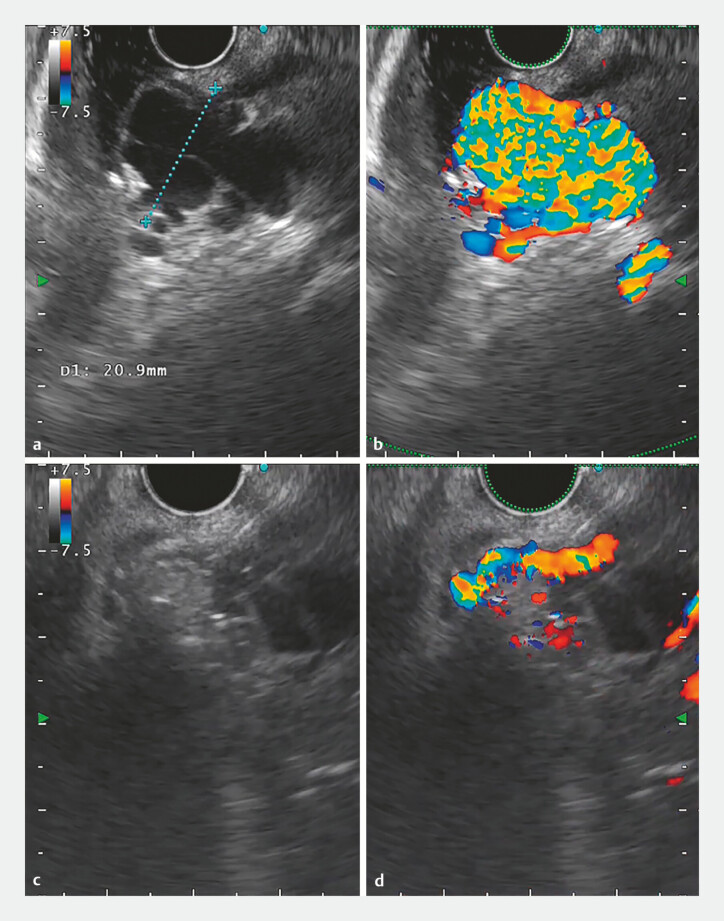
Endoscopic ultrasound (EUS) images showing the rectal varices:
